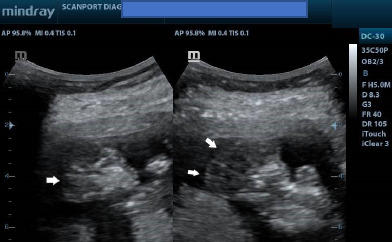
Ultrasound images showing sagittal and parasagittal images of the brain demonstrating the absence of the calvaria. See the margins of the brain (white arrows) without bony margins
